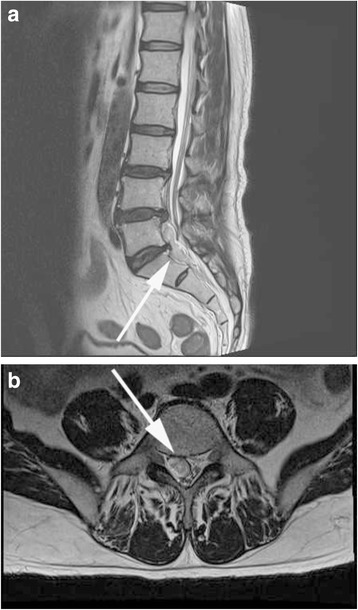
a T2WI sagittal plane MR image showing an epidural hematoma extending from L4 to S1 level. b T2WI axial MR image showing the epidural hematoma
